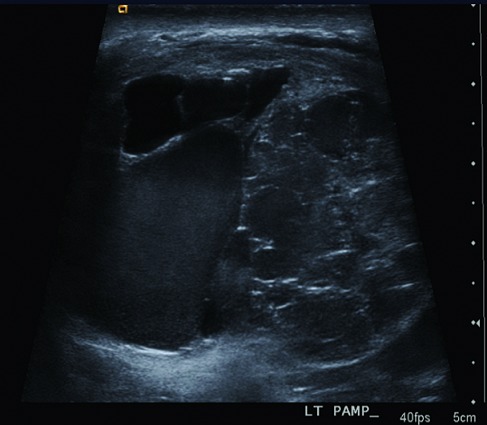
Scrotal ultrasound shows mixed solid and multilocular cystic mass, containing cysts of varying echogenicties
